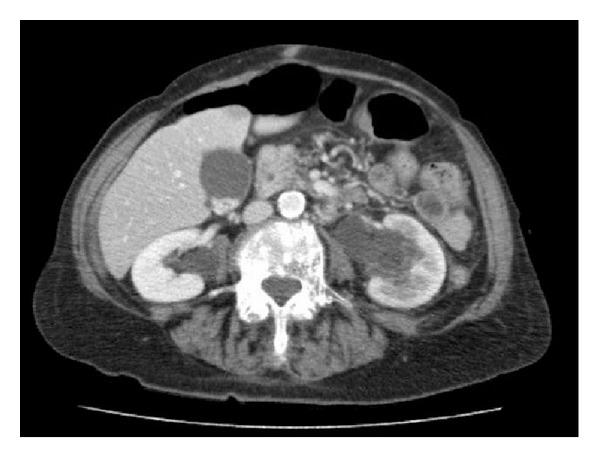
Abdominal and Pelvic CT showing bilateral hydronephrosis.
